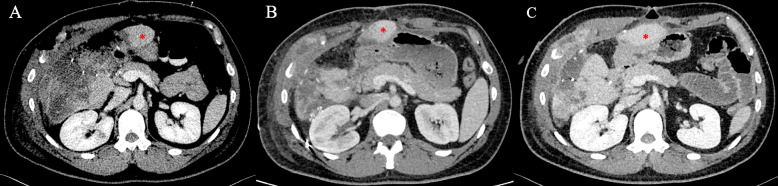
CT-scans that demonstrate improvement in perfusion in damaged segments of the liver
